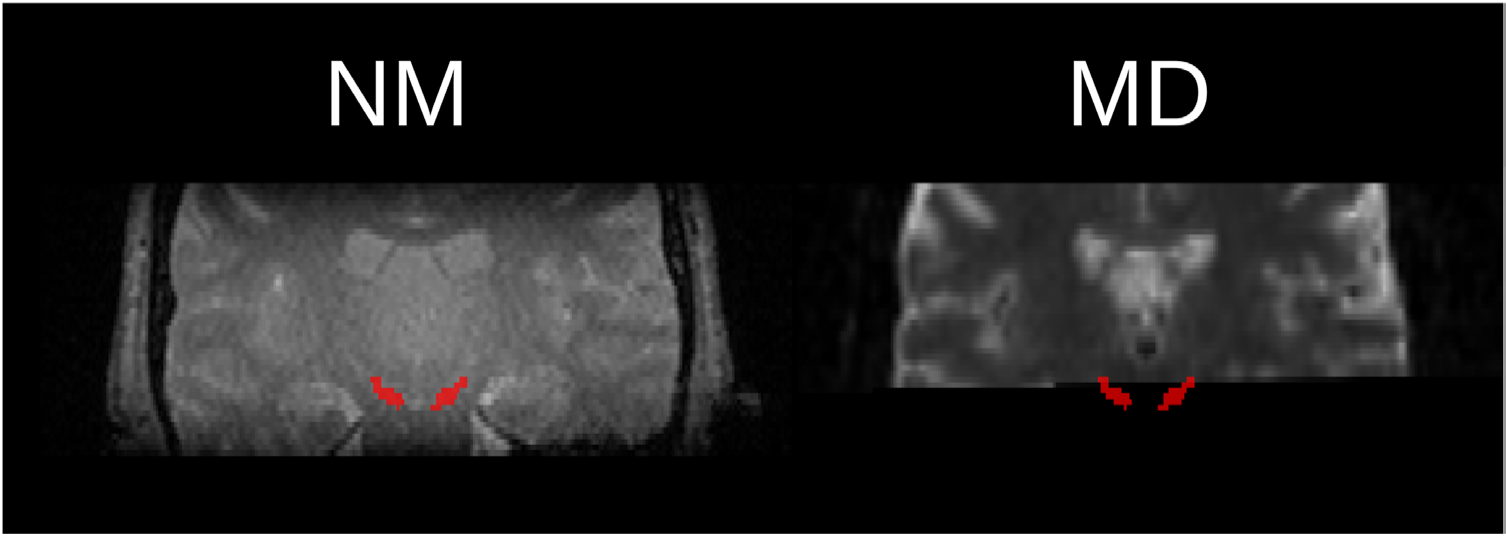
Extreme case showing NM-MRI (left) and MD map (mean diffusivity, right) from the same participant and slice, demonstrating a poor coverage of the SN (red) in the MD.
